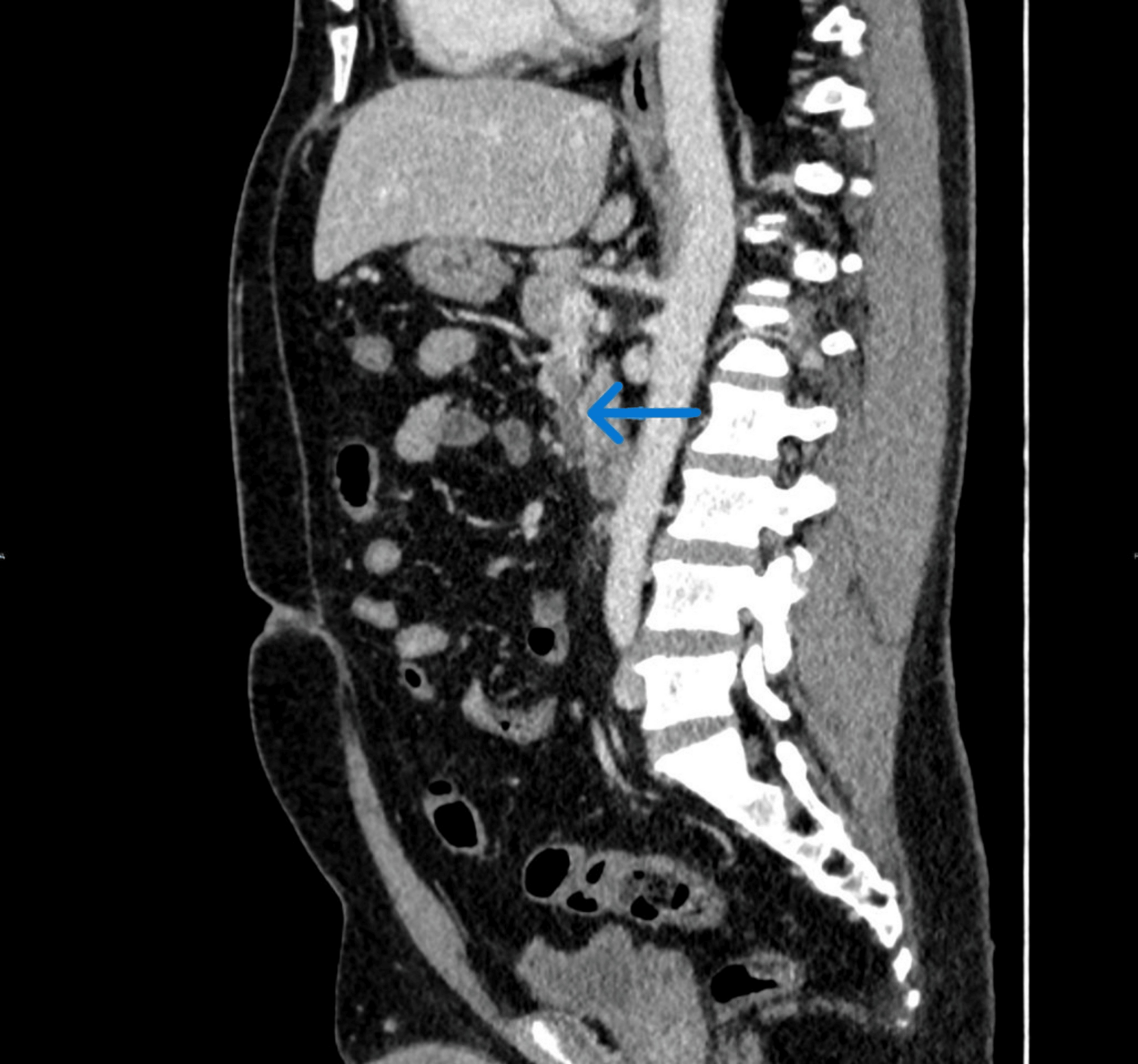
Sagittal CT abdomen showing SMVT A CT scan of the abdomen in sagittal view reveals a filling defect in the SMV, consistent with thrombosis. The blue arrow indicates the site of the thrombus. SMV, superior mesenteric vein; SMVT, superior mesenteric vein thrombosis
